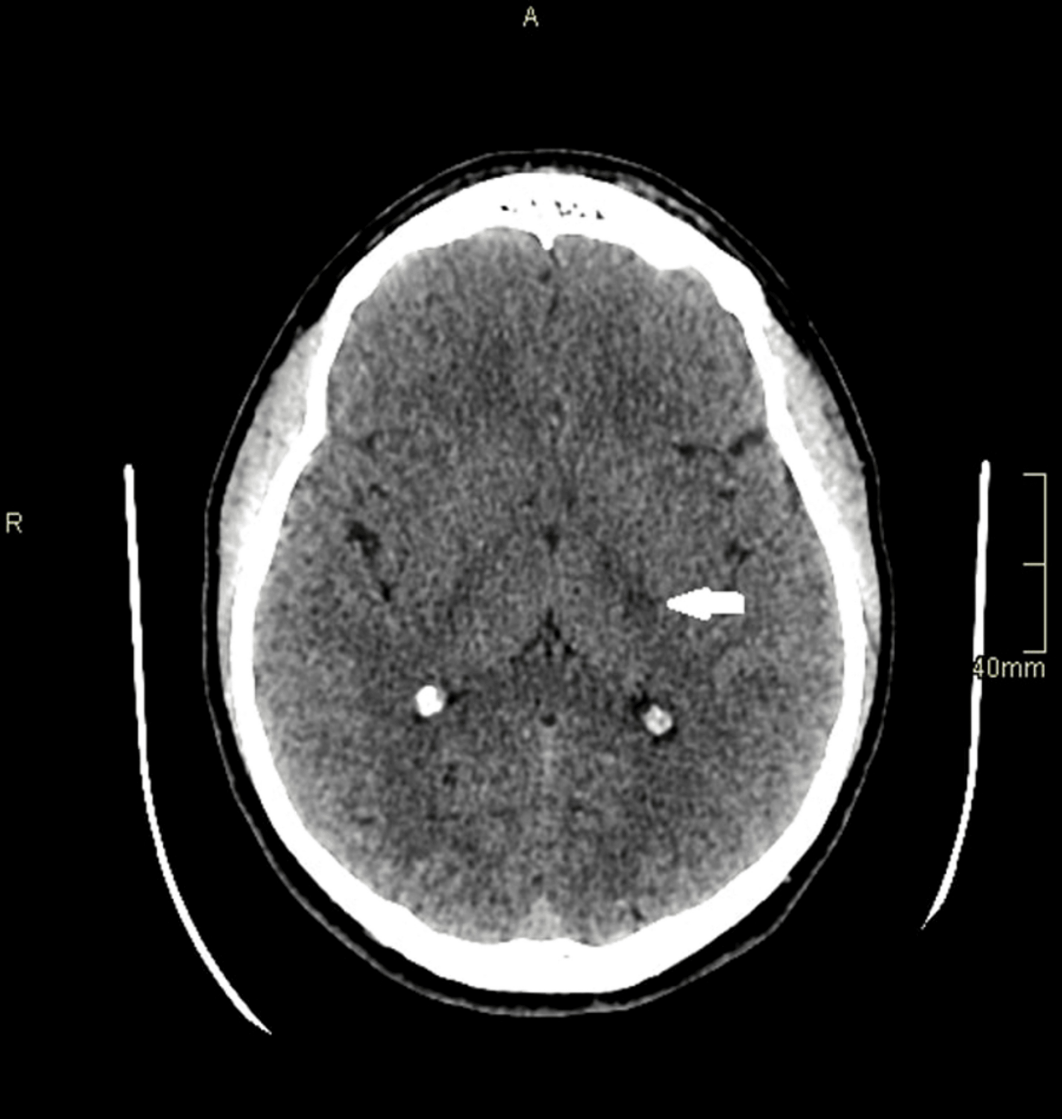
Non-contrast CT revealing left capsulo-thalamic hypodensity
